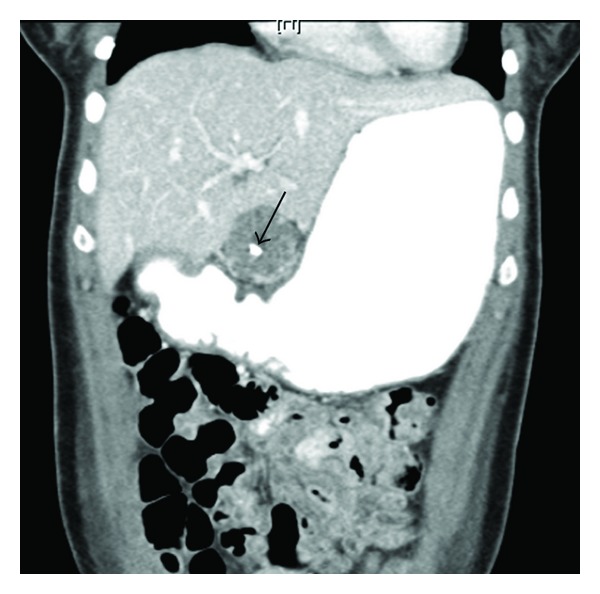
Serous cystadenoma with central scar (arrow) on abdominal CT.
